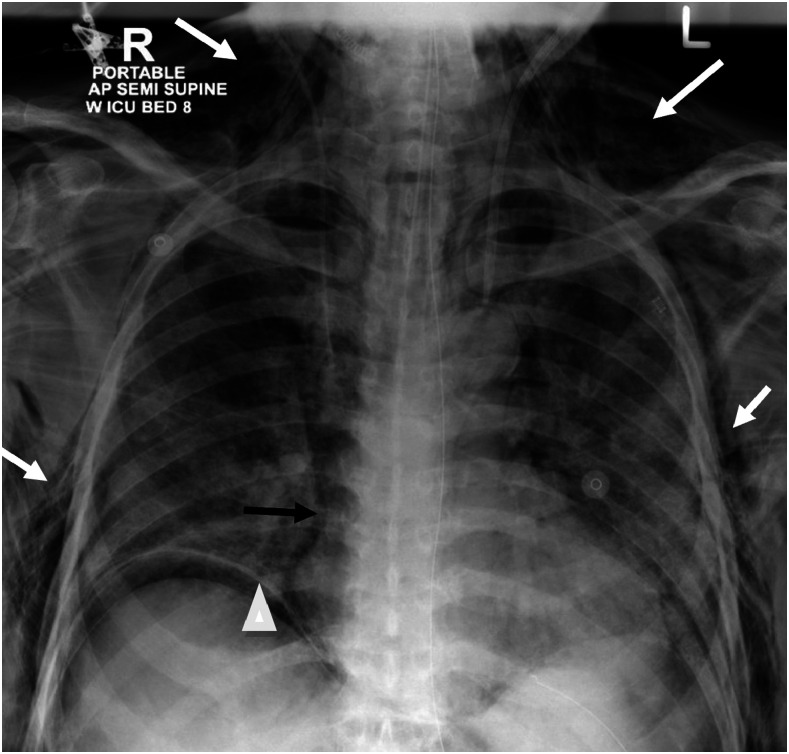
Chest X-ray examination showing surgical emphysema (white arrows), pneumomediastinum (black arrows), and pneumoperitoneum indicated by air under the diaphragm (arrowhead).
